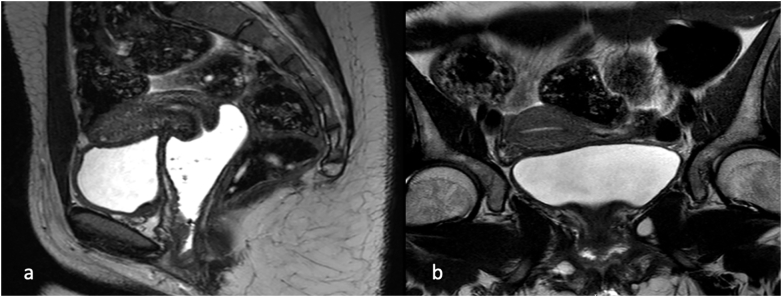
(a-b) MRI of the pelvis without evidence of oncologic disease.
